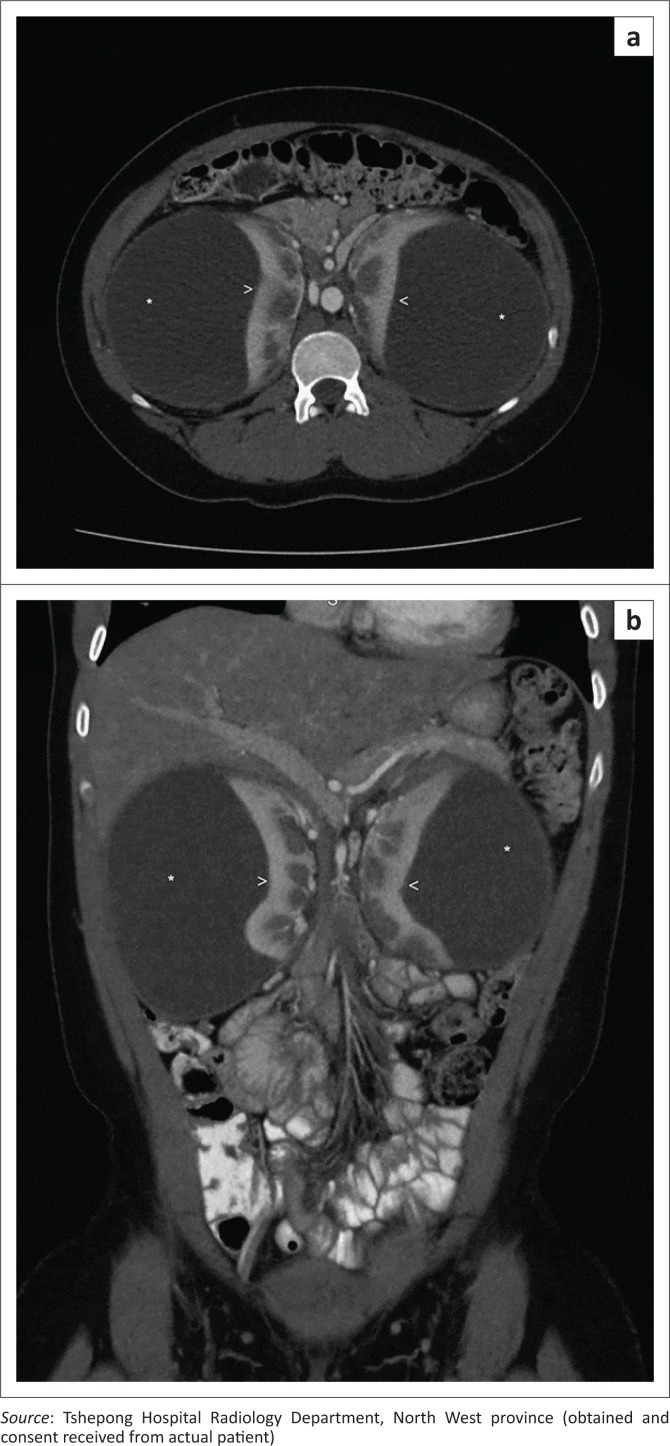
(a, b) Contrast-enhanced axial and coronal computed tomography of the abdomen demonstrating large bilateral subcapsular collections (stars) causing compression of the renal parenchyma (arrowheads).
